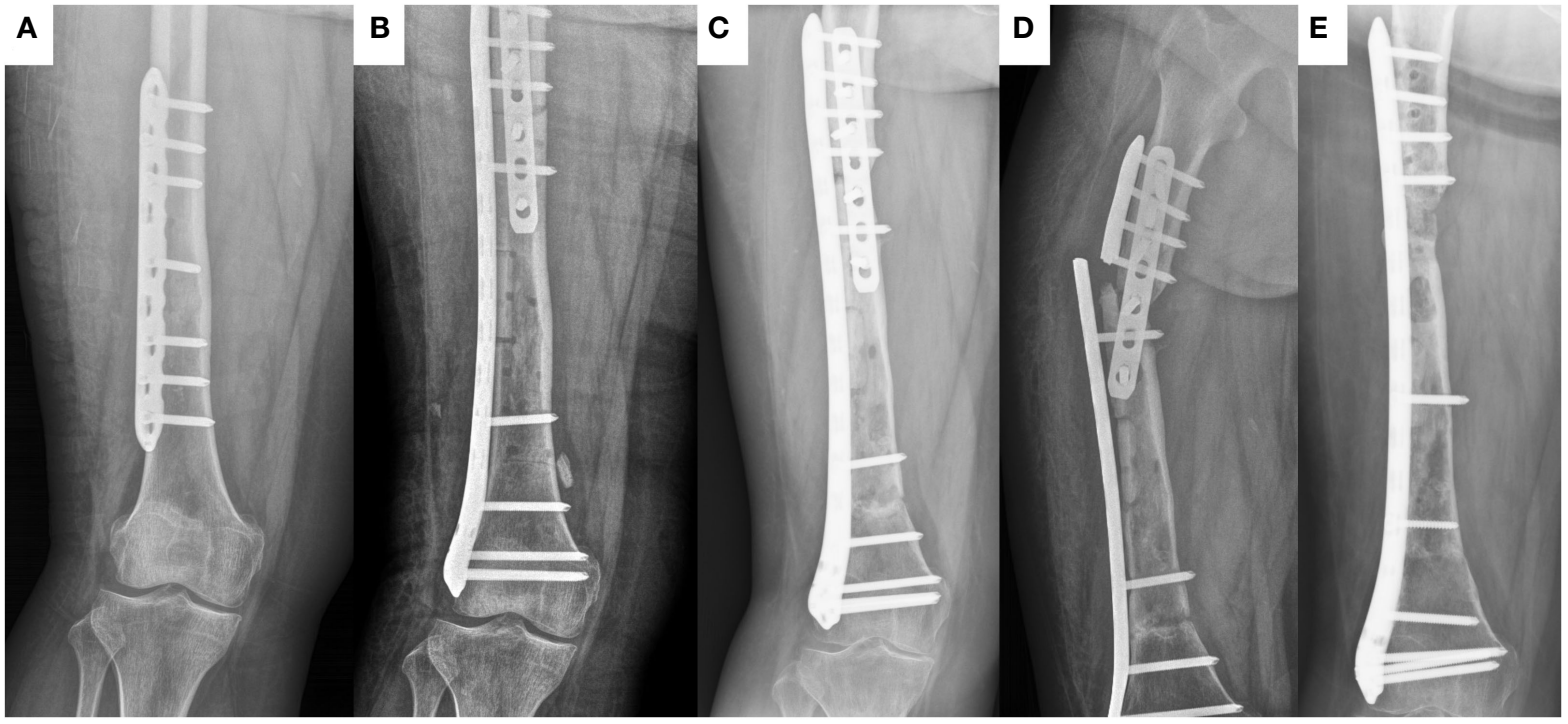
The healing process of frozen inactivated autologous tumor bone in a 15-year old female (case 37) with Ewing sarcoma involving the diaphysis of the left femur. (A) preoperative X-ray. (B) postoperative X-ray. (C) X-ray at 18 months postoperation. (D) X-ray at 20 months postoperation (At this point, the plate breaks). (E) X-ray at 11 months after last operation.
